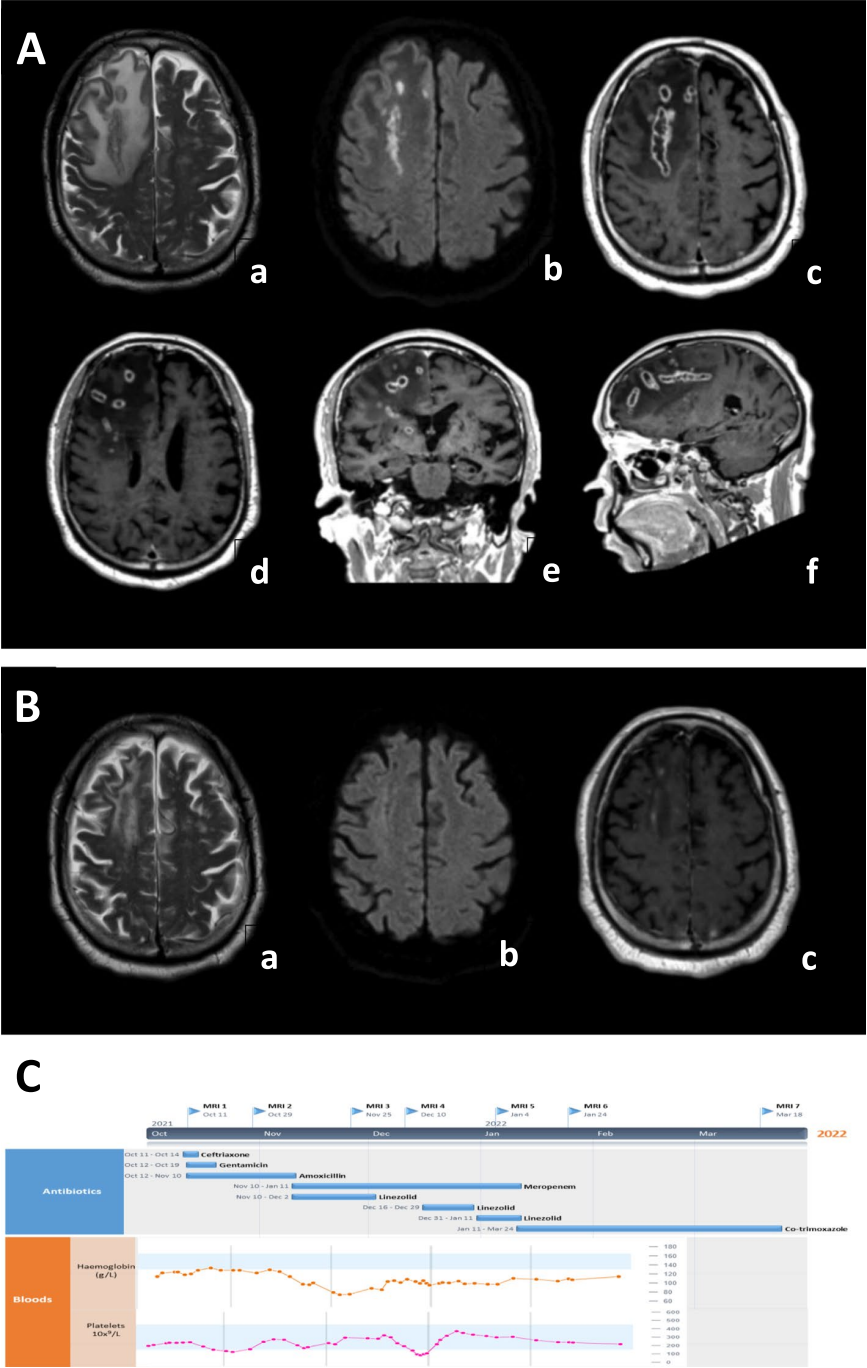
MRI scans of the brain at different stages of disease and timeline of antibiotic therapy. A. Initial MRI scan of the brain: axial T2-weighted image (a), axial diffusion-weighted image (b), and axial (c and d), coronal (d) and sagittal (e and f) post-gadolinium T1-weighted images. There are multiple linear and ovoid rim enhancing lesions in the right frontal lobe with a “tunnel sign” (arrows) and coalescence of smaller lesions (arrowheads). There is surrounding white matter T2 hyperintensity indicating vasogenic oedema and central diffusion restriction, seen as high signal, within all of the lesions suggestive of purulent content. This appearance is diagnostic for multiple bacterial abscesses, the morphology is suggestive of *Listeria sp*. B. Post treatment MRI brain scan at 5 months. Axial T2-weighted (a), axial diffusion-weighted (b) and post-gadolinium axial T1-weighted images (c) demonstrate residual mild oedema in the right frontal lobe, no diffusion restriction and minimal contrast enhancement. C. Antibiotic timeline in relation to timing of serial MRI brain imaging during the 125 days of hospitalisation. The patient was finally discharged at the end of February 2022 on oral co-trimoxazole. Timeline of platelets and haemoglobin in relation to antibiotics shows the transient linezolid-induced bone marrow toxicity
